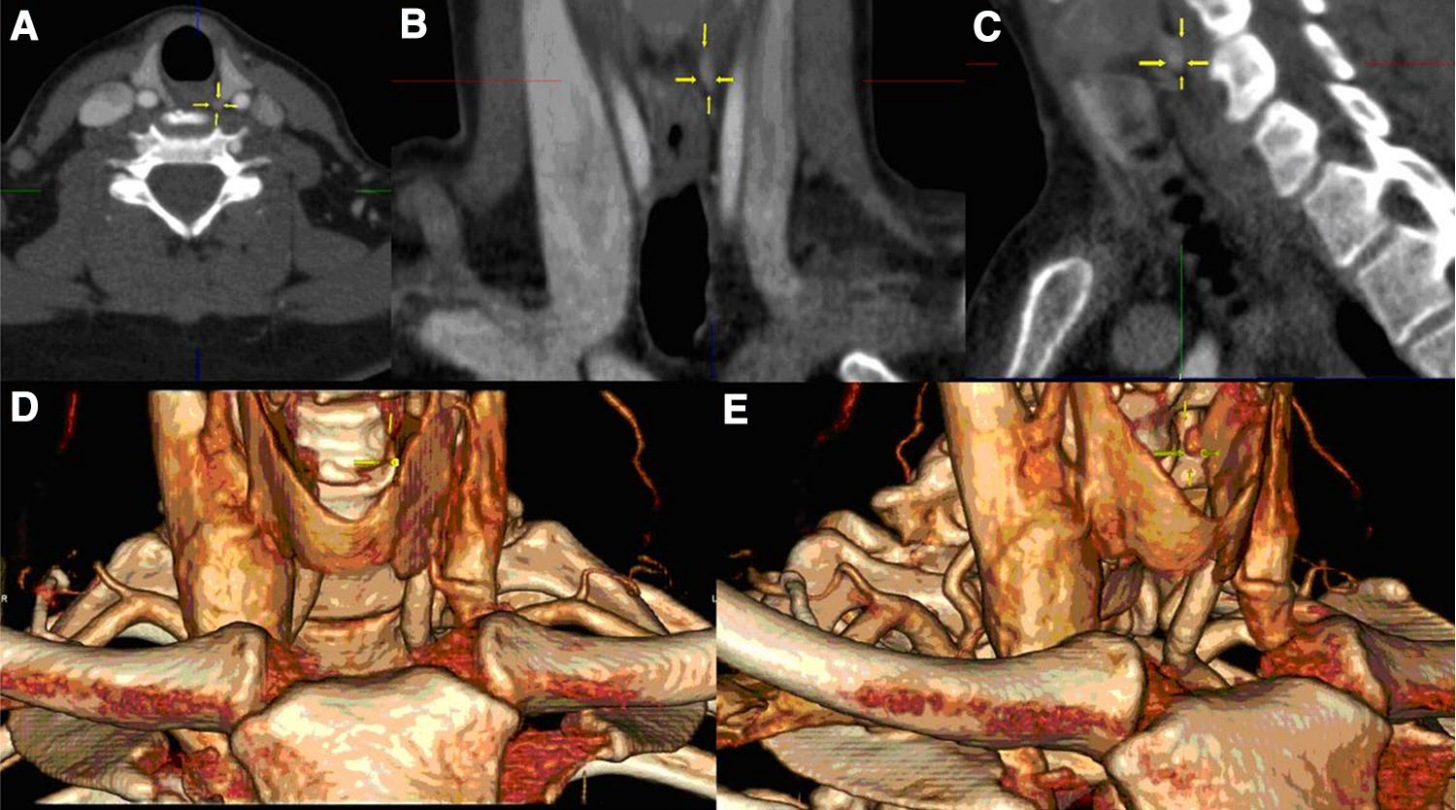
Example of preoperative localization of pathological parathyroid tissue with 4D-CT scan. Demonstration of an area suspicious for a parathyroid adenoma (*yellow arrows*) in the *left upper* quadrant in a axial, b coronal and c sagittal plane, and d, e three-dimensional reconstruction
